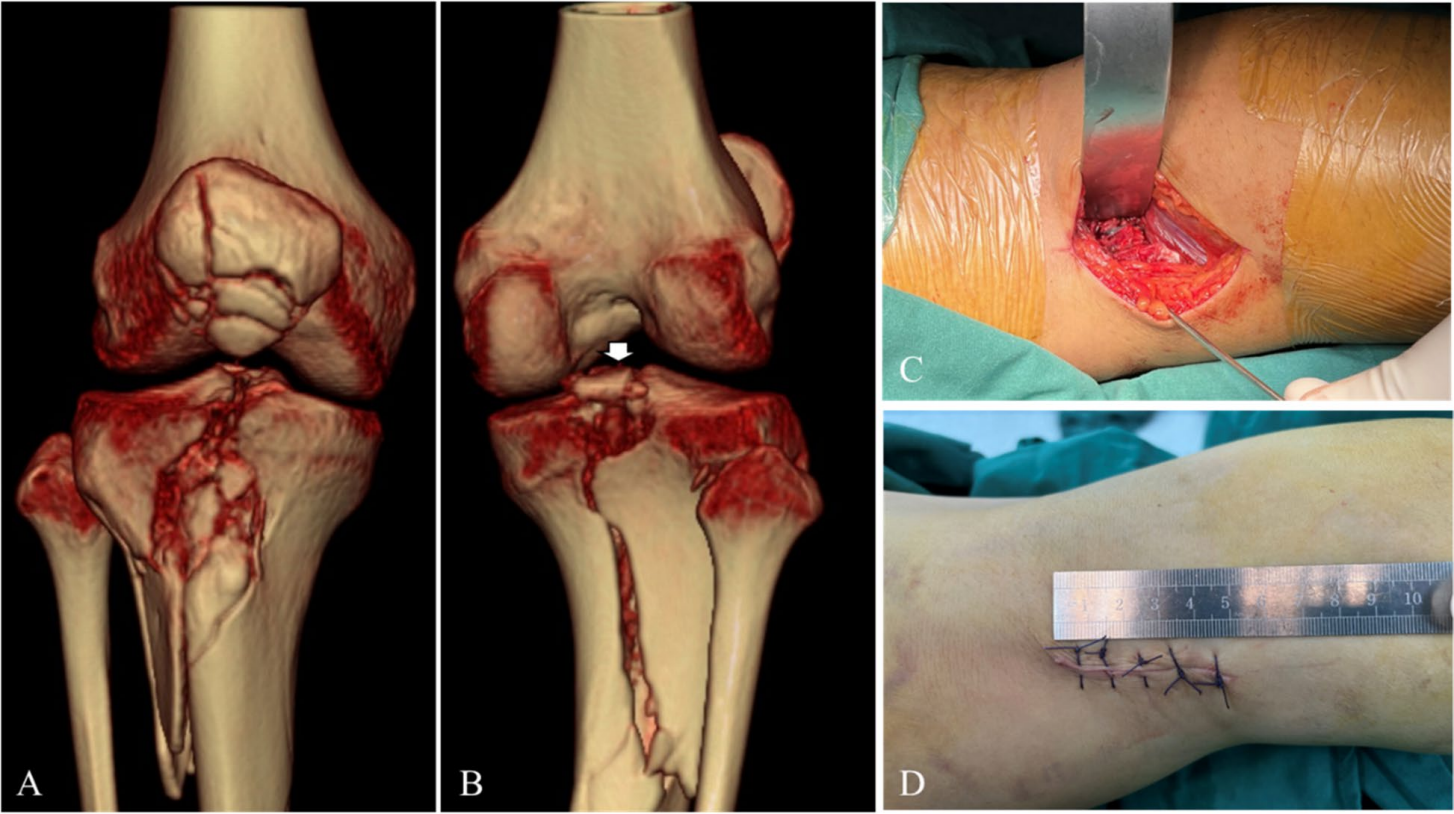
Preoperative knee three-dimensional (3D) computed tomography (CT): anterior (A) and posterior (B) views showed patellar comminuted, proximal tibial comminuted and PCL tibial avulsion fractures. The intraoperative incision pictures of the minimally invasive posteromedial approach showed the exposure of the operative site (C) with a 5 cm length incision (D)
